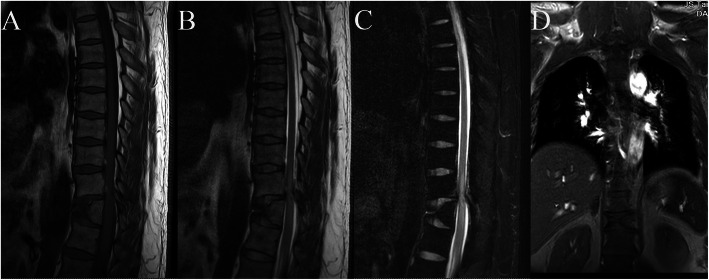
Magnetic resonance imaging (MRI) of the spine after injury. T1-weighted MRI (a) shows normalized signal intensities of the spinal cord. T2-weighted (b) and fat-suppressed MRI sequences (c) show a high-signal lesion in the spinal cord adjacent to the T11 vertebra. A coronal image (d) shows the presence of a butterfly vertebra at the T11 level. There was no signal change in the vertebral bodies or intervertebral discs
